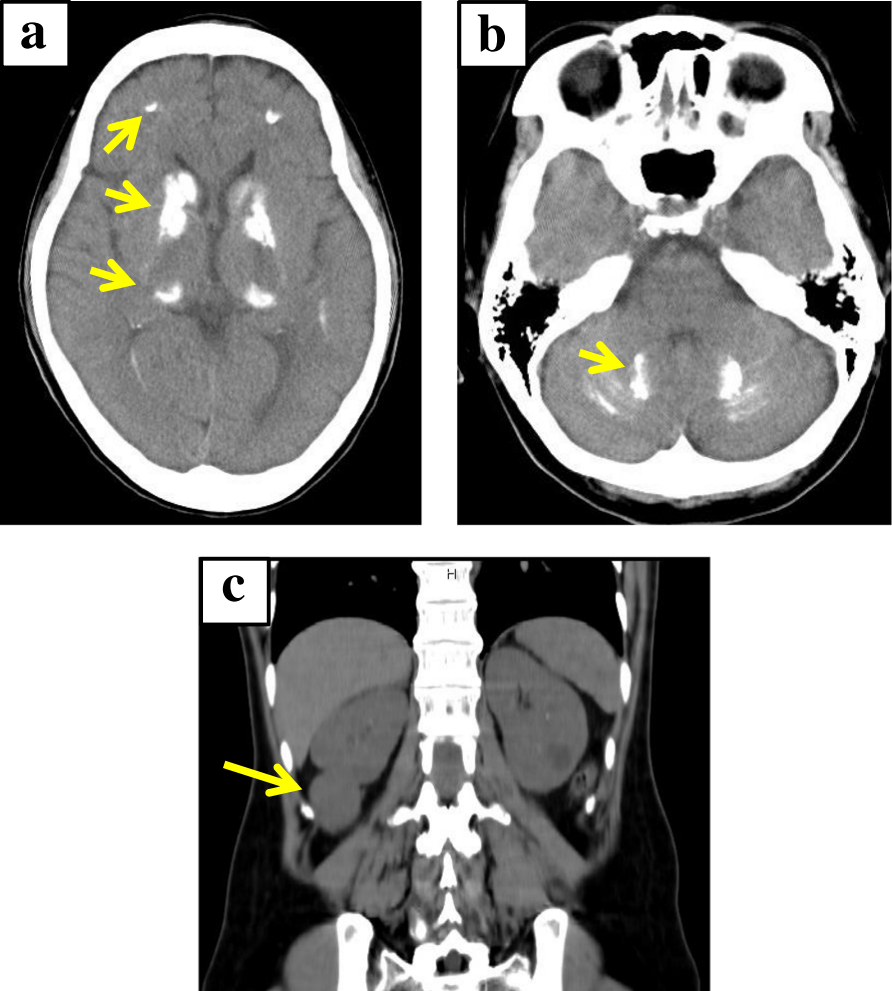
Plain head and abdominal computed tomography (CT) scans of the proband. a, b. Head CT. Severe calcification was observed in the basal ganglia, thalamus, deep white matter (a), and cerebellum (b) (arrow). c. Abdominal CT. Renal dysplasia can be seen on the right side (arrow)
